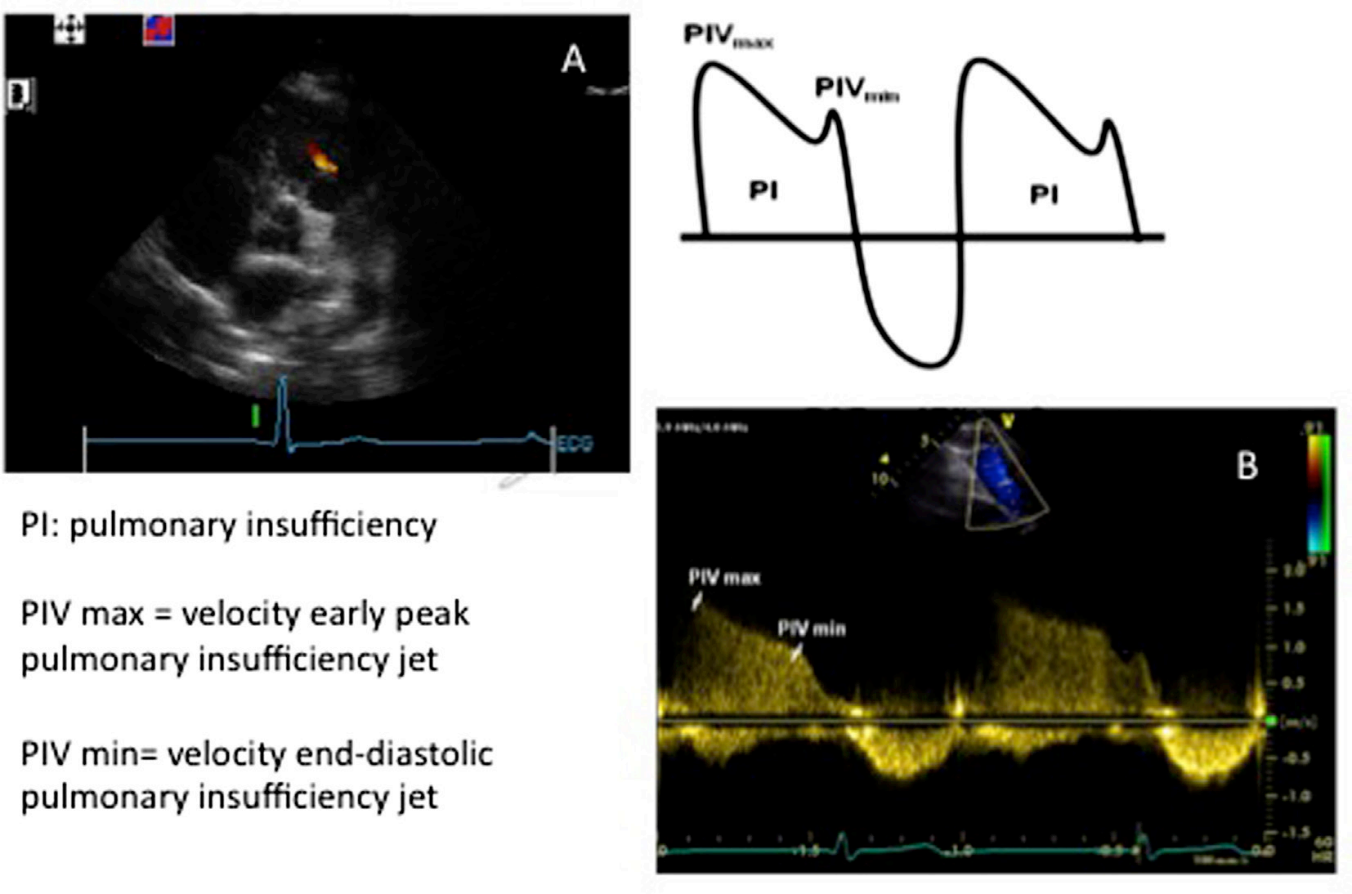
Images showing (A) short axis view and a pulmonary insufficiency jet and (B) Doppler envelope of the pulmonary insufficiency jet allowing for measurement of the peak velocity of the pulmonary insufficiency jet (PIV_max_) and the peak velocity of the end diastolic pulmonary insufficiency jet (PIV_min_). See the text for formulas.
